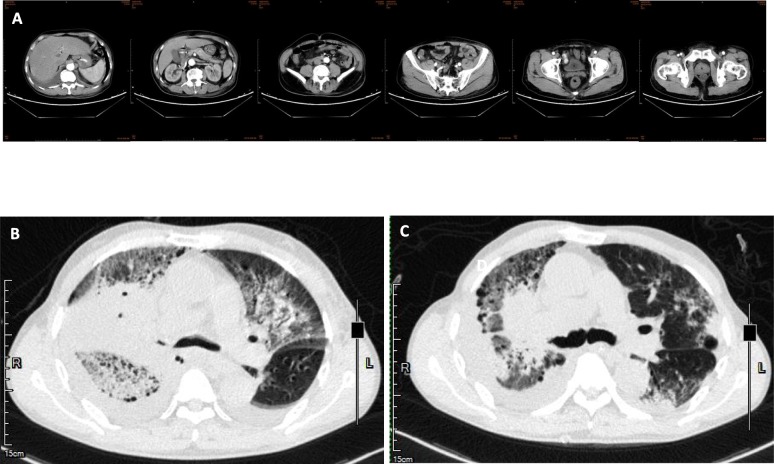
Chest CT results of *Acrophialophora* infection. a no abnormal images were found in the enhanced CT scan of the whole abdomen on September 5, 2018. b Chest CT images prior to treatment. c Chest CT images after treatment with liposomal amphotericin B
